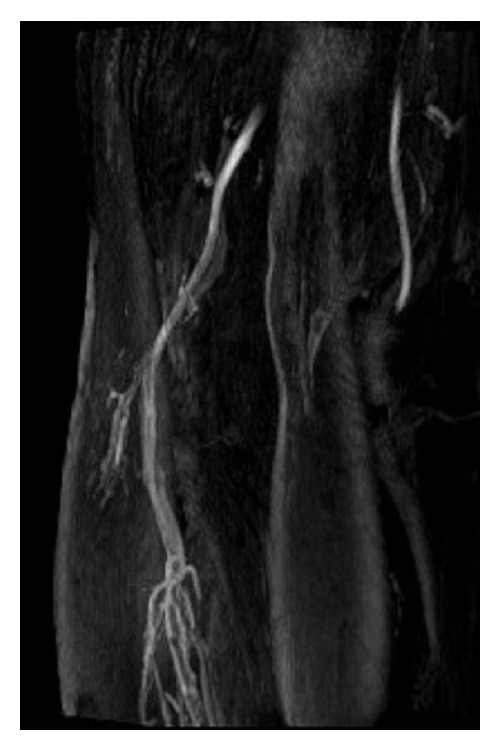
MRI angiogram of the popliteal fossa showing complete occlusion of the popliteal artery in the left leg.
